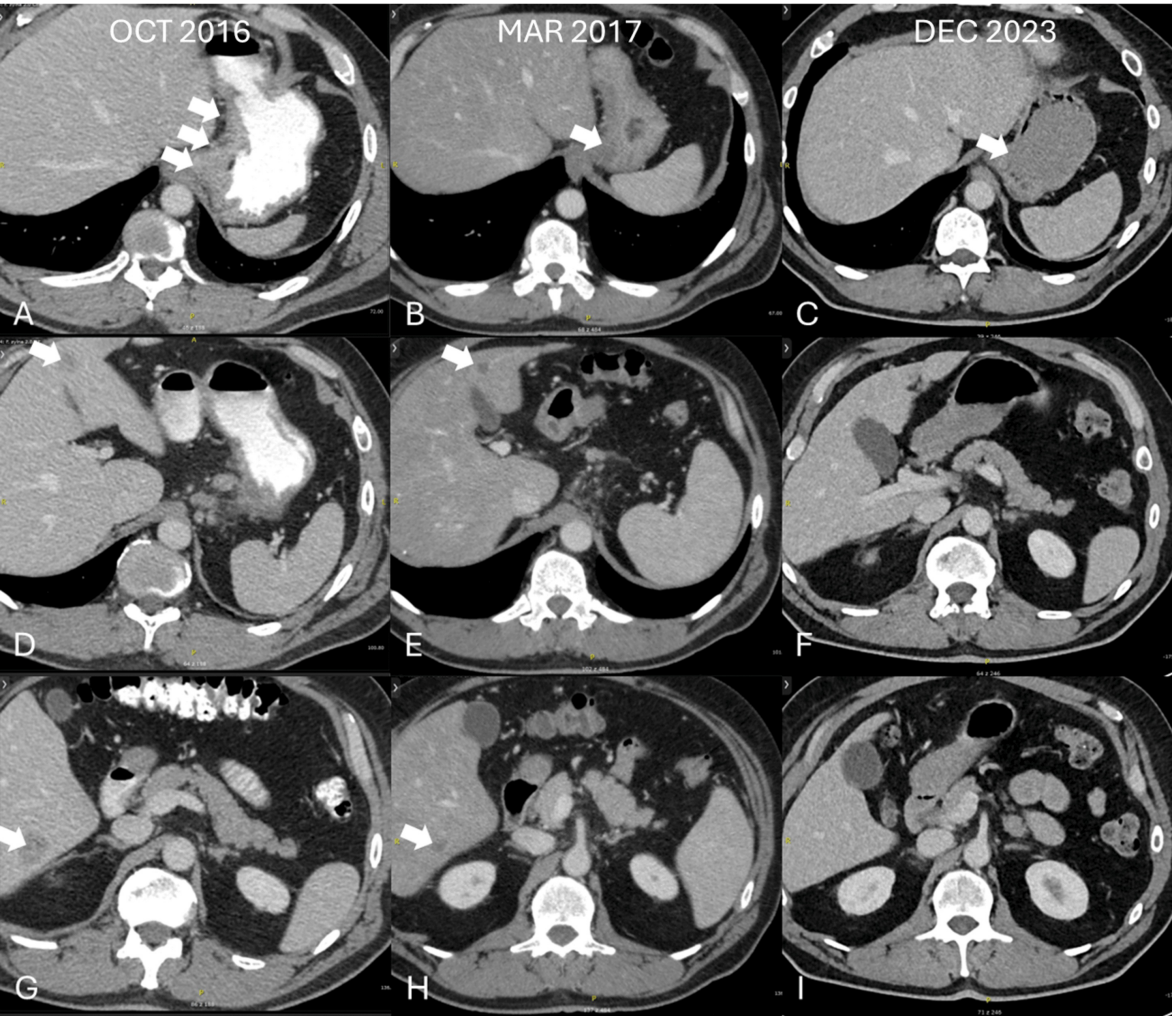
CT scans of representative cancer lesions at three timepoints. (A) The primary lesion at baseline (October 2016): contrast enhancing infiltrated cardia and enlarged regional lymph nodes; (B) the primary lesion after four months of therapy (March 2017): remission of the cardia infiltration; (C) the primary lesion at the last available follow-up scan (December 2023): no visible pathology. (D) The liver lesion in segment 4b at baseline (October 2016): peripheral contrast enhancement and hypodense center; (E) the liver lesion in segment 4b after four months of therapy (March 2017): diminished peripheral contrast enhancement and decreased diameter; (F) the liver lesion in segment 4b at the last available follow-up scan (December 2023): no visible pathology. (G) The liver lesion in segment 6 at baseline (October 2016): peripheral contrast enhancement and hypodense center; (H) the liver lesion in segment 6 after four months of therapy (March 2017): diminished peripheral contrast enhancement and decreased diameter; (I) the liver lesion in segment 6 at the last available follow-up scan (December 2023): no visible pathology. All scans presented were captured in the venous phase.
